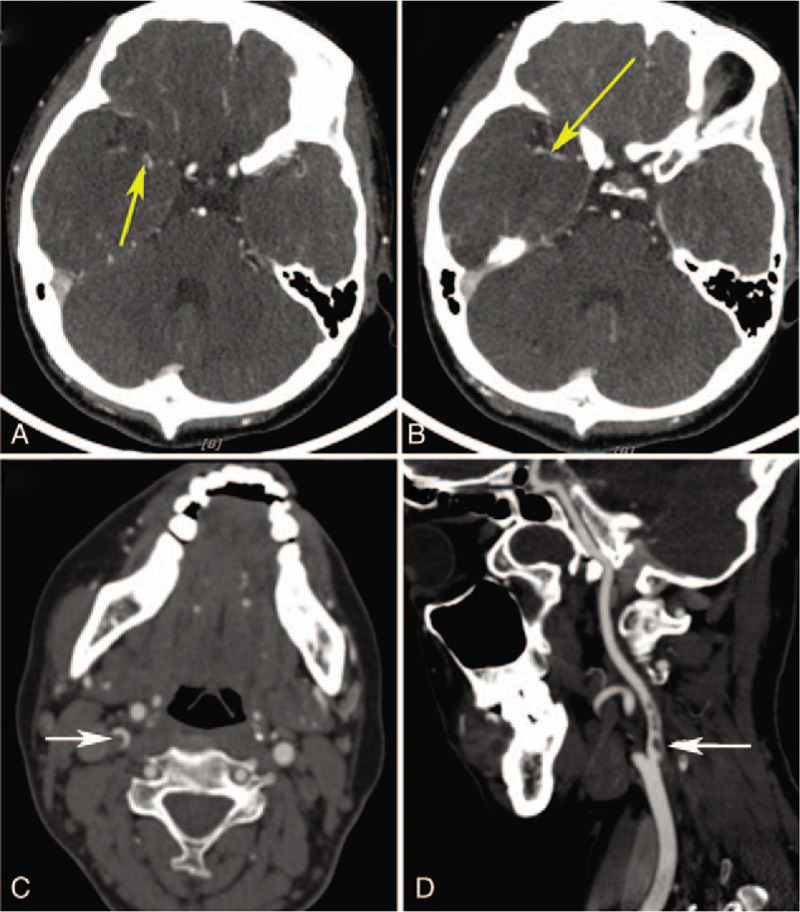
Computed tomographic angiography (CTA) of the brain and neck (A, B: the cerebral angiography; C, D: the cervical angiography). The CTA revealed an occlusion of the distal segment in the right cerebral middle artery (yellow arrow) and a 21.5% stenosis of the right internal carotid artery (ICA) (white arrow).
